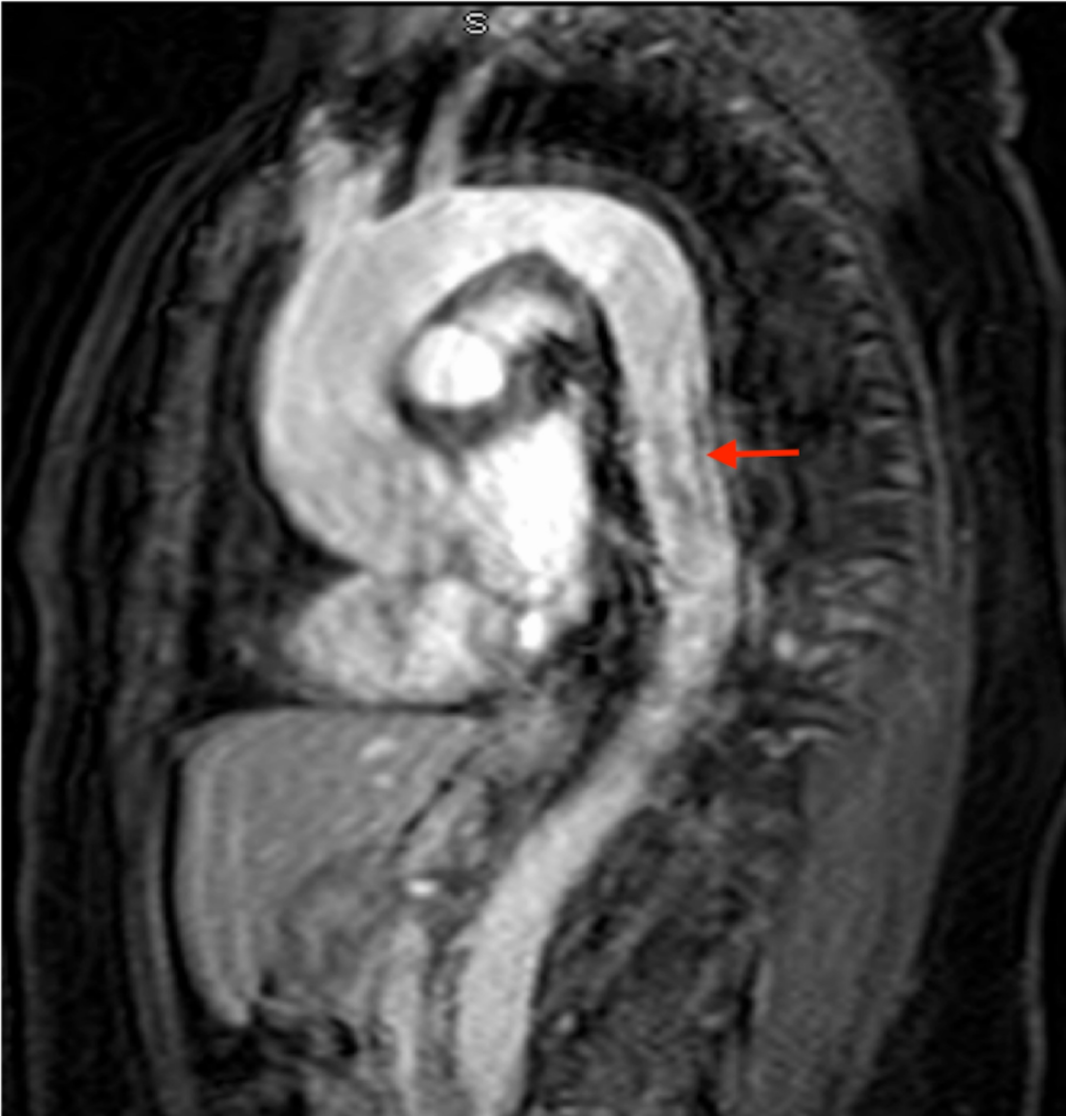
Coronal MRI of the aortic wall The red arrow indicates necrosis in the aortic wall due to aortitis. MRI: magnetic resonance imaging
